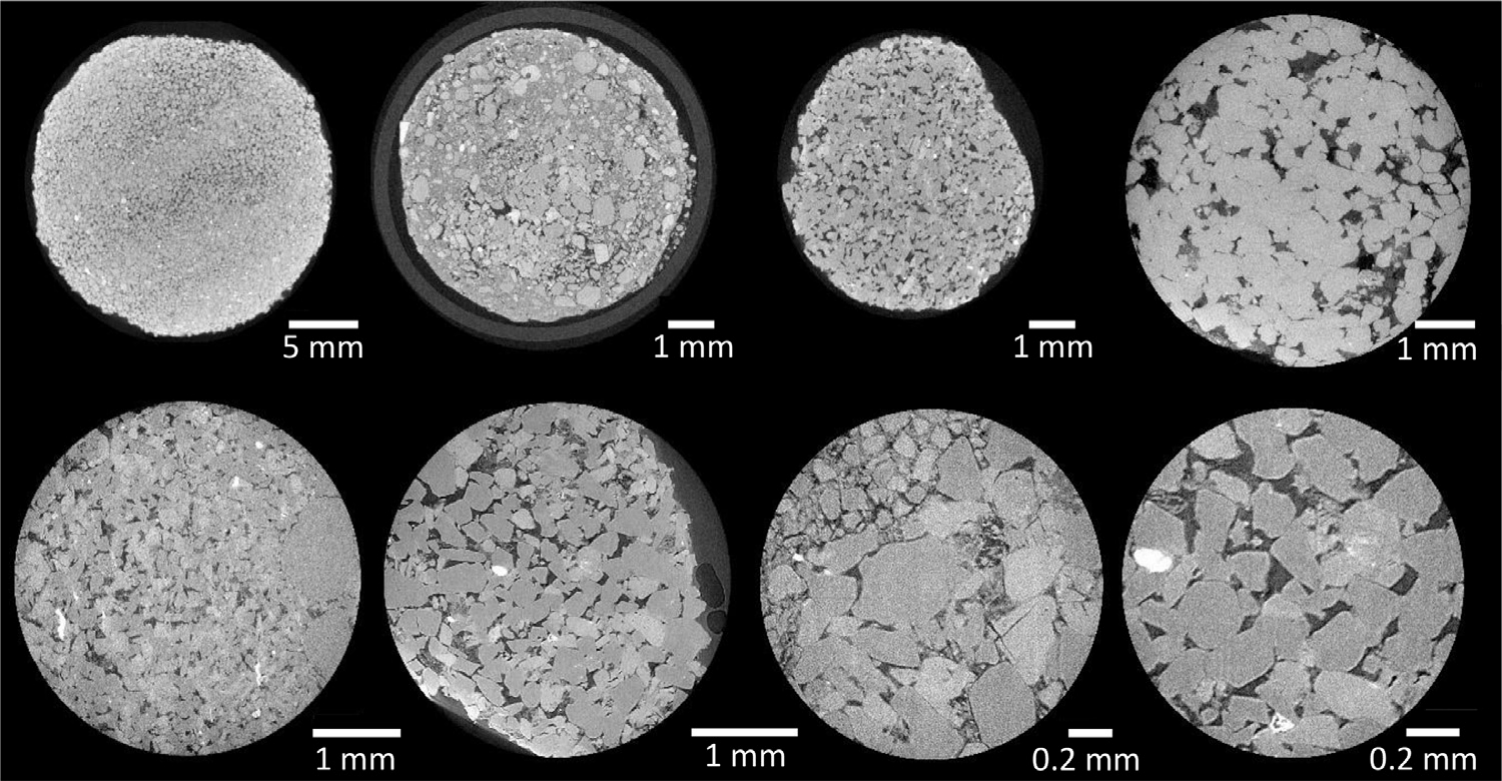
Representative computed tomography slices from a selection of available scans. From left to right: Top row from: 1-Mt.Simon_6986ft_Dry_IndCT, 1-Mt.Simon_VW1_6919.3-6926.6ft_M70, 1-Mt.Simon_6927ft_DryScan_M70, Mt.Simon_VW2_4x_7054.5; and Bottom row: 8-Mt.Simon_VW1_6919.3-6926.6ft_4x_AreaB, 12-Mt.Simon_VW1_6919.3-6926.6ft_Epoxy_4x, 2-Mt.Simon_VW1_6919.3-6926.6ft_10x, 14-Mt.Simon_VW1_6919.3-6926.6ft_Epoxy_10x.
